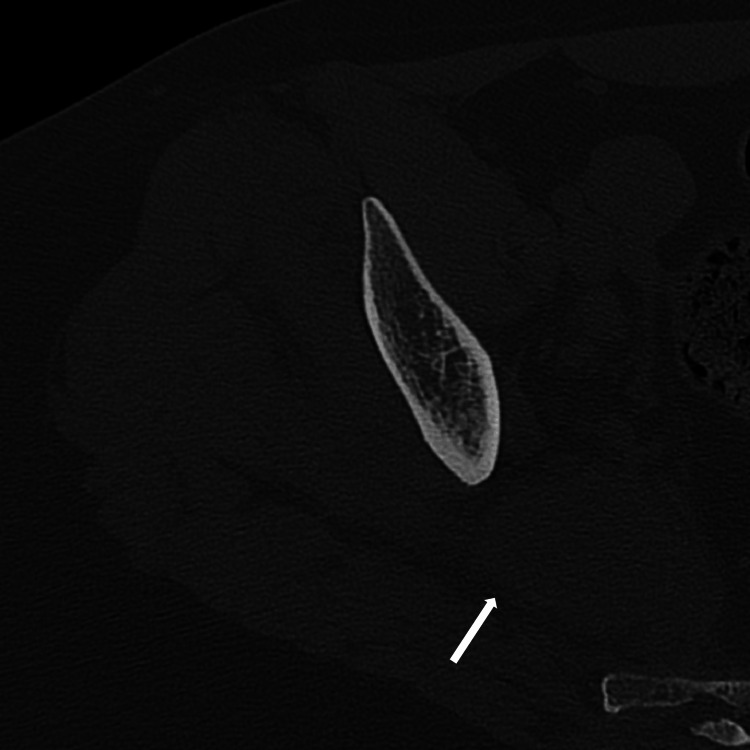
A CT of the right hip taken at urgent care demonstrates abnormal fluid collection in the right piriformis.
